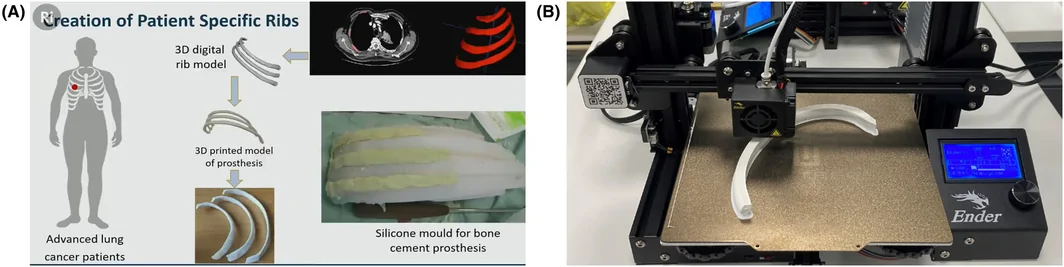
Design of patient‐specific ribs with computer modeling and 3D printing. (A) Design process of synthetic ribs, from computer modeling based on patient CT scans to manufacturing. Image taken from Dr. Pontiki's presentation at the Royal Institution. (B) 3D printing of a prototype model in progress.
